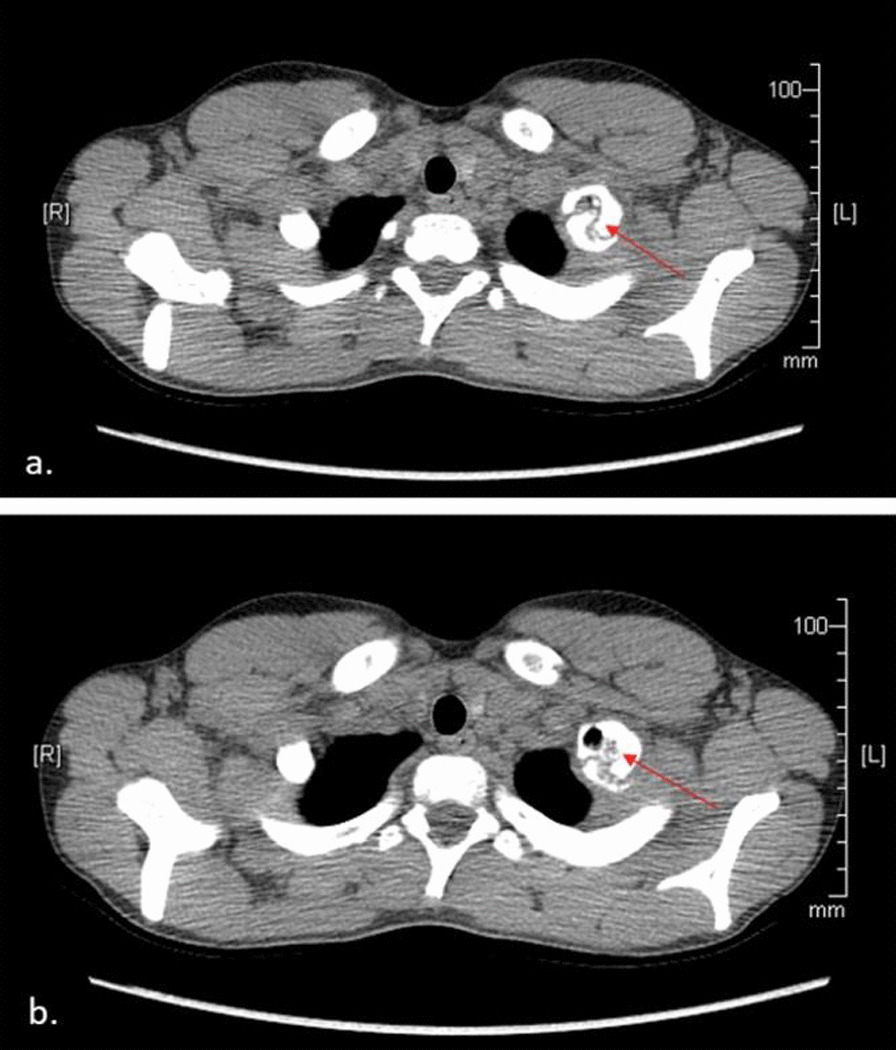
a, b Axial computed tomography (CT) scan revealing pseudoarthrosis of left first rib with vacuum phenomenon (red arrow is pointing to the pseudoarthrosis present and the vacuum effect on the first rib)
